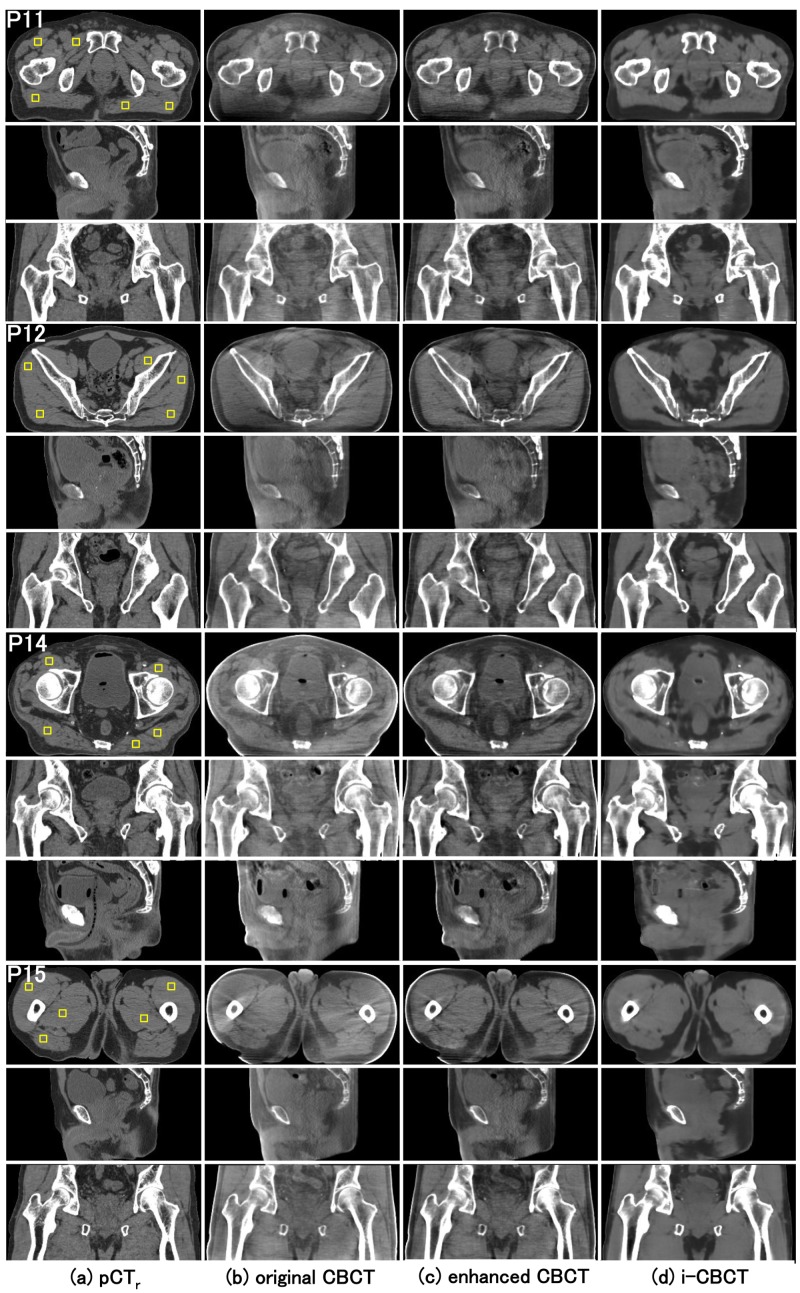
Comparison of  the image qualities for another four cases (patient 11, 12, 14, 15). For each patient, the images in the top, middle, and bottom row are axial, sagittal and coronal views, respectively. Columns (a), (b), (c) and (d) are pCT_r_, original CBCT, enhanced CBCT and i-CBCT, respectively. The yellow squares placed on the axial view of the column (a) were selected ROIs for the SNU calculation. The ROIs were set on the muscle region for each patient. Display window range was set to (-400, 100) HU for the original CBCT and (-300, 200) HU for the pCT_r_, the enhanced CBCT and the i-CBCT. pCT_r_; Registered planning computed tomography; original CBCT: Original cone beam computed tomography; enhanced-CBCT: Enhanced cone beam computed tomography; i-CBCT: Improved cone beam computed tomography; ROI: Region of interest; SNU: Spatial nonuniformity.
